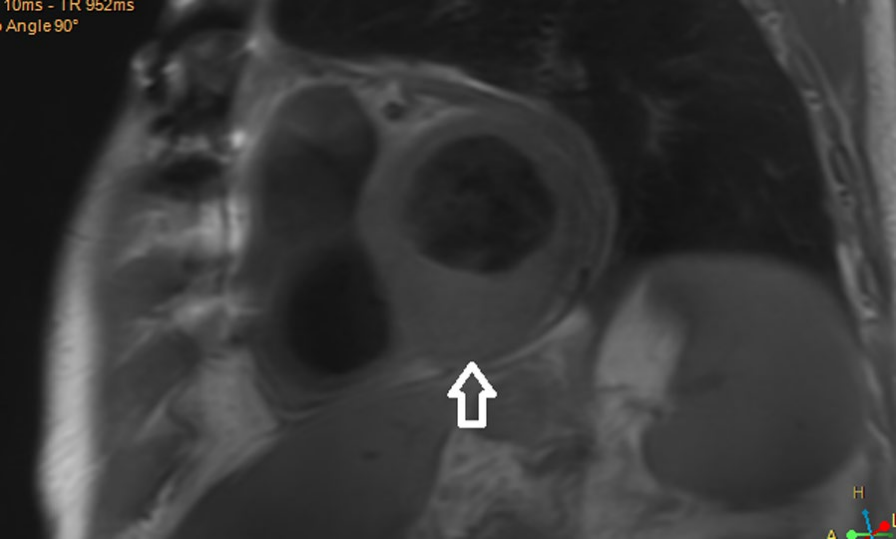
Cardiac Magnetic Resonance Imaging: T1-weighted image in short axis view revealing an isointense lesion at the base of the LV (indicated by arrow)
